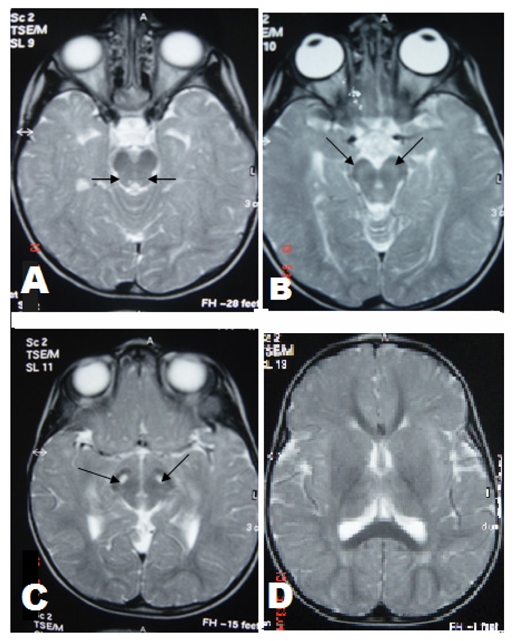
(A-C): Axial T2-weighted images through the brainstem show symmetrical hyperintense lesions involving central tegmental tracts (thin arrow in A), substantia nigra (thin arrow in B and C). (D): Axial T2-weighted image through basal ganglia shows putamen, globus pallidus and thalamus were not involved.
